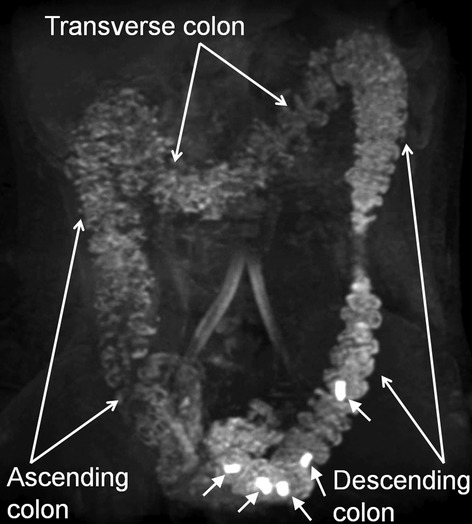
Maximum intensity projection (MIP) magnetic resonance imaging (MRI) image. Showing five MRI marker capsules in the colon (indicated by close arrows). Images created using water only images acquired using the T1 weighted multi-echo FFE pulse sequence. Such images were used to locate the number and position of capsules remaining at 24 h to calculate a whole gut transit time.
